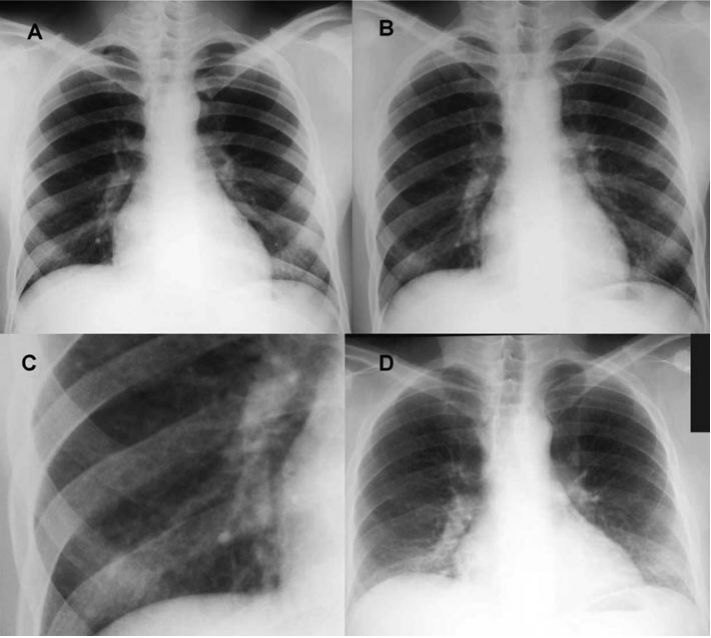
Chest radiographs of case 1. (*A*) Radiograph taken pre hire on 2 March 1979, showing normal lung fields. (*B* ) Radiograph taken during the second episode of acute work-related illness on 17 March 1981, showing a mild diffuse nodular infiltrate; (*C*) close-up of the right lower lung field from radiograph in (*B*). (*D* ) Radiograph at follow-up on 13 February 1997, showing reduced lung volumes and a bilateral interstitial infiltrate.
